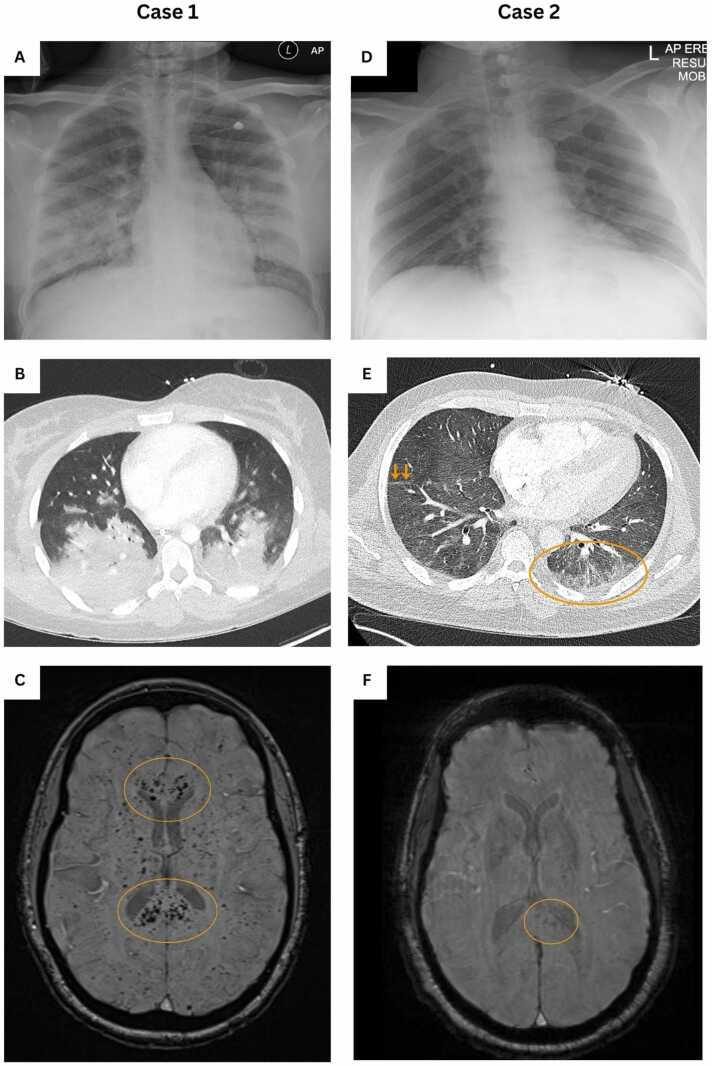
Imaging of the chest and brain for the patients in cases 1 and 2. Case 1: (A) Chest radiograph: bilateral mid-zone airspace opacification. (B) Axial computed tomography (CT) images of the thorax, lung window: bilateral severe consolidation. (C) Axial magnetic resonance (MR) images of the brain, susceptibility weighted imaging (SWI) sequence: innumerable punctate foci of susceptibility within both cerebral hemispheres, most densely within the corpus callosum (circled). Case 2: (D) Chest radiograph: no focal consolidation, collapse, or effusion. (E) Axial CT images of the thorax, lung window: ground-glass opacification in the left lower lobe (circled) and linear atelectasis (arrows). (F) Axial MR images of the brain, SWI sequence, degraded by motion artefact: punctate foci of susceptibility, less diffuse than in Case 1, most evident in the splenium of the corpus callosum, to the left of midline (circled).
